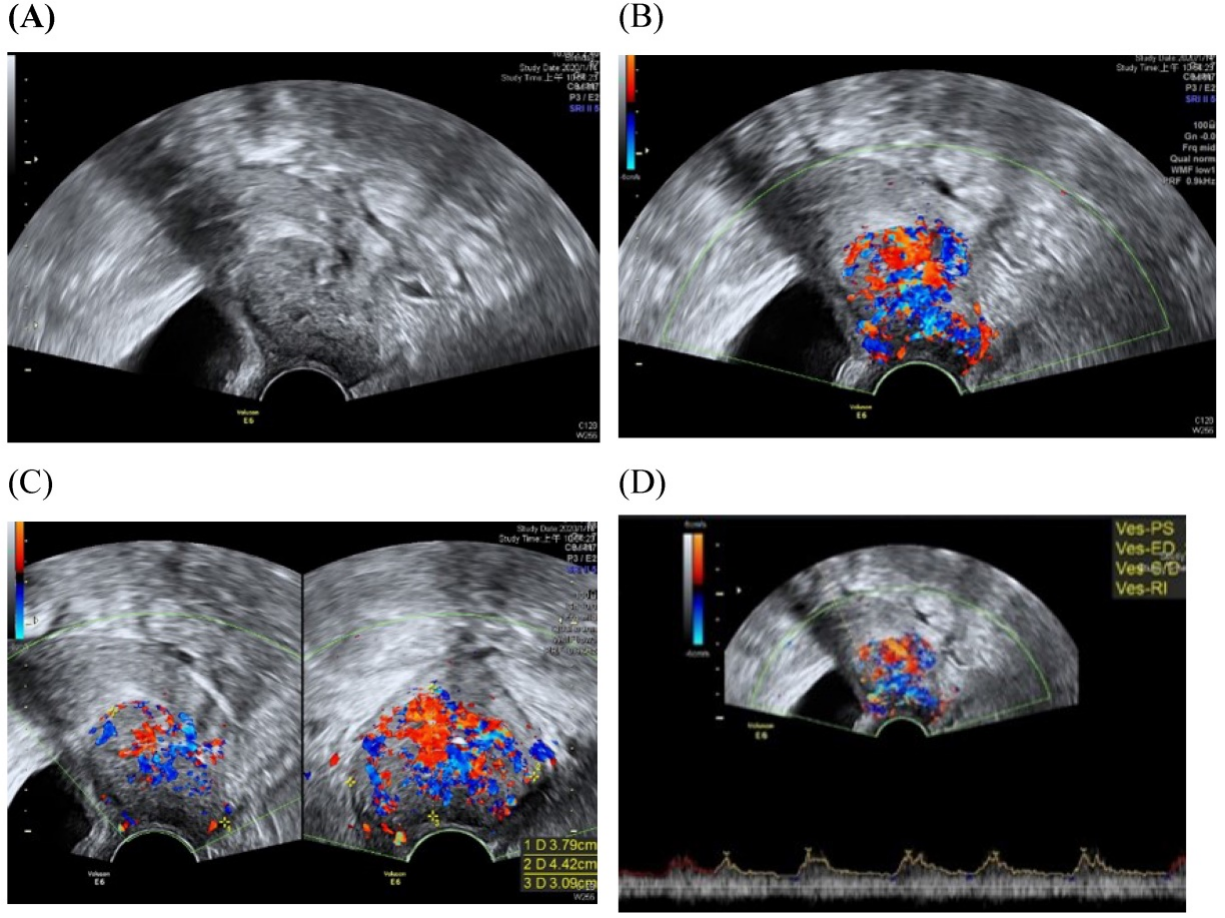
Transvaginall ultrasound image gray scale and color Doppler shows a longitudinal section of the uterus with cervical cancer of the female aged 68-year-old. (A) Gray scale shows cervical mass (B) color Doppler shows abundant blood flow in cervical mass (C) color Doppler (D) color Doppler Blood flow.
